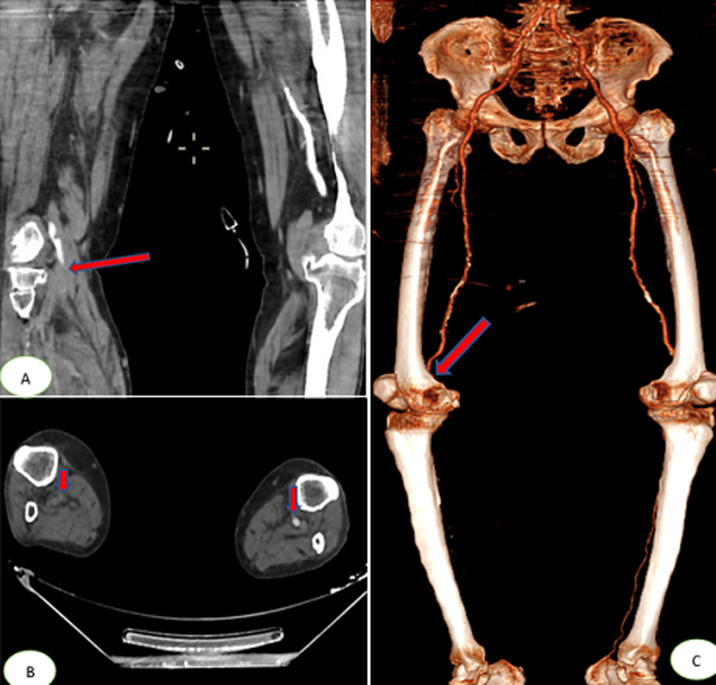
(A,B,C) computed tomography angiography of the lower limbs showing an abrupt arrest in the right popliteal artery (red arrows)
